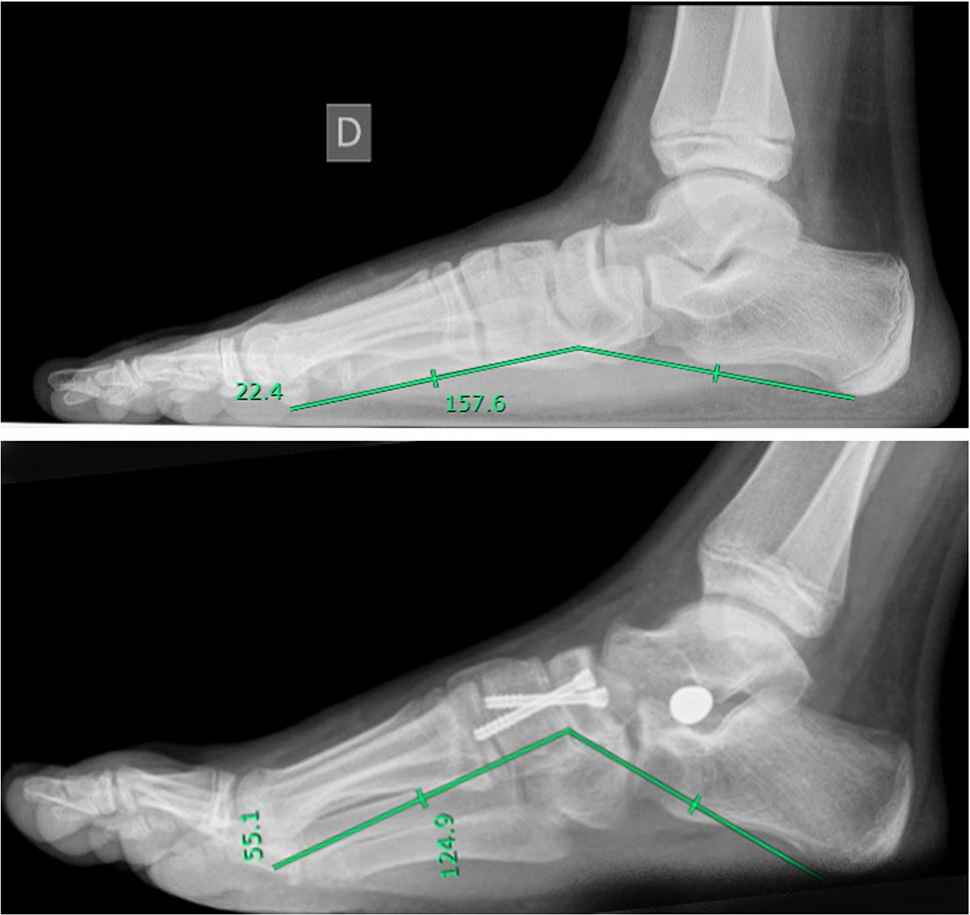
Evaluation of Costa-Bertani angle in a pre (left panel) and post (right panel) surgical x-ray in a right foot
